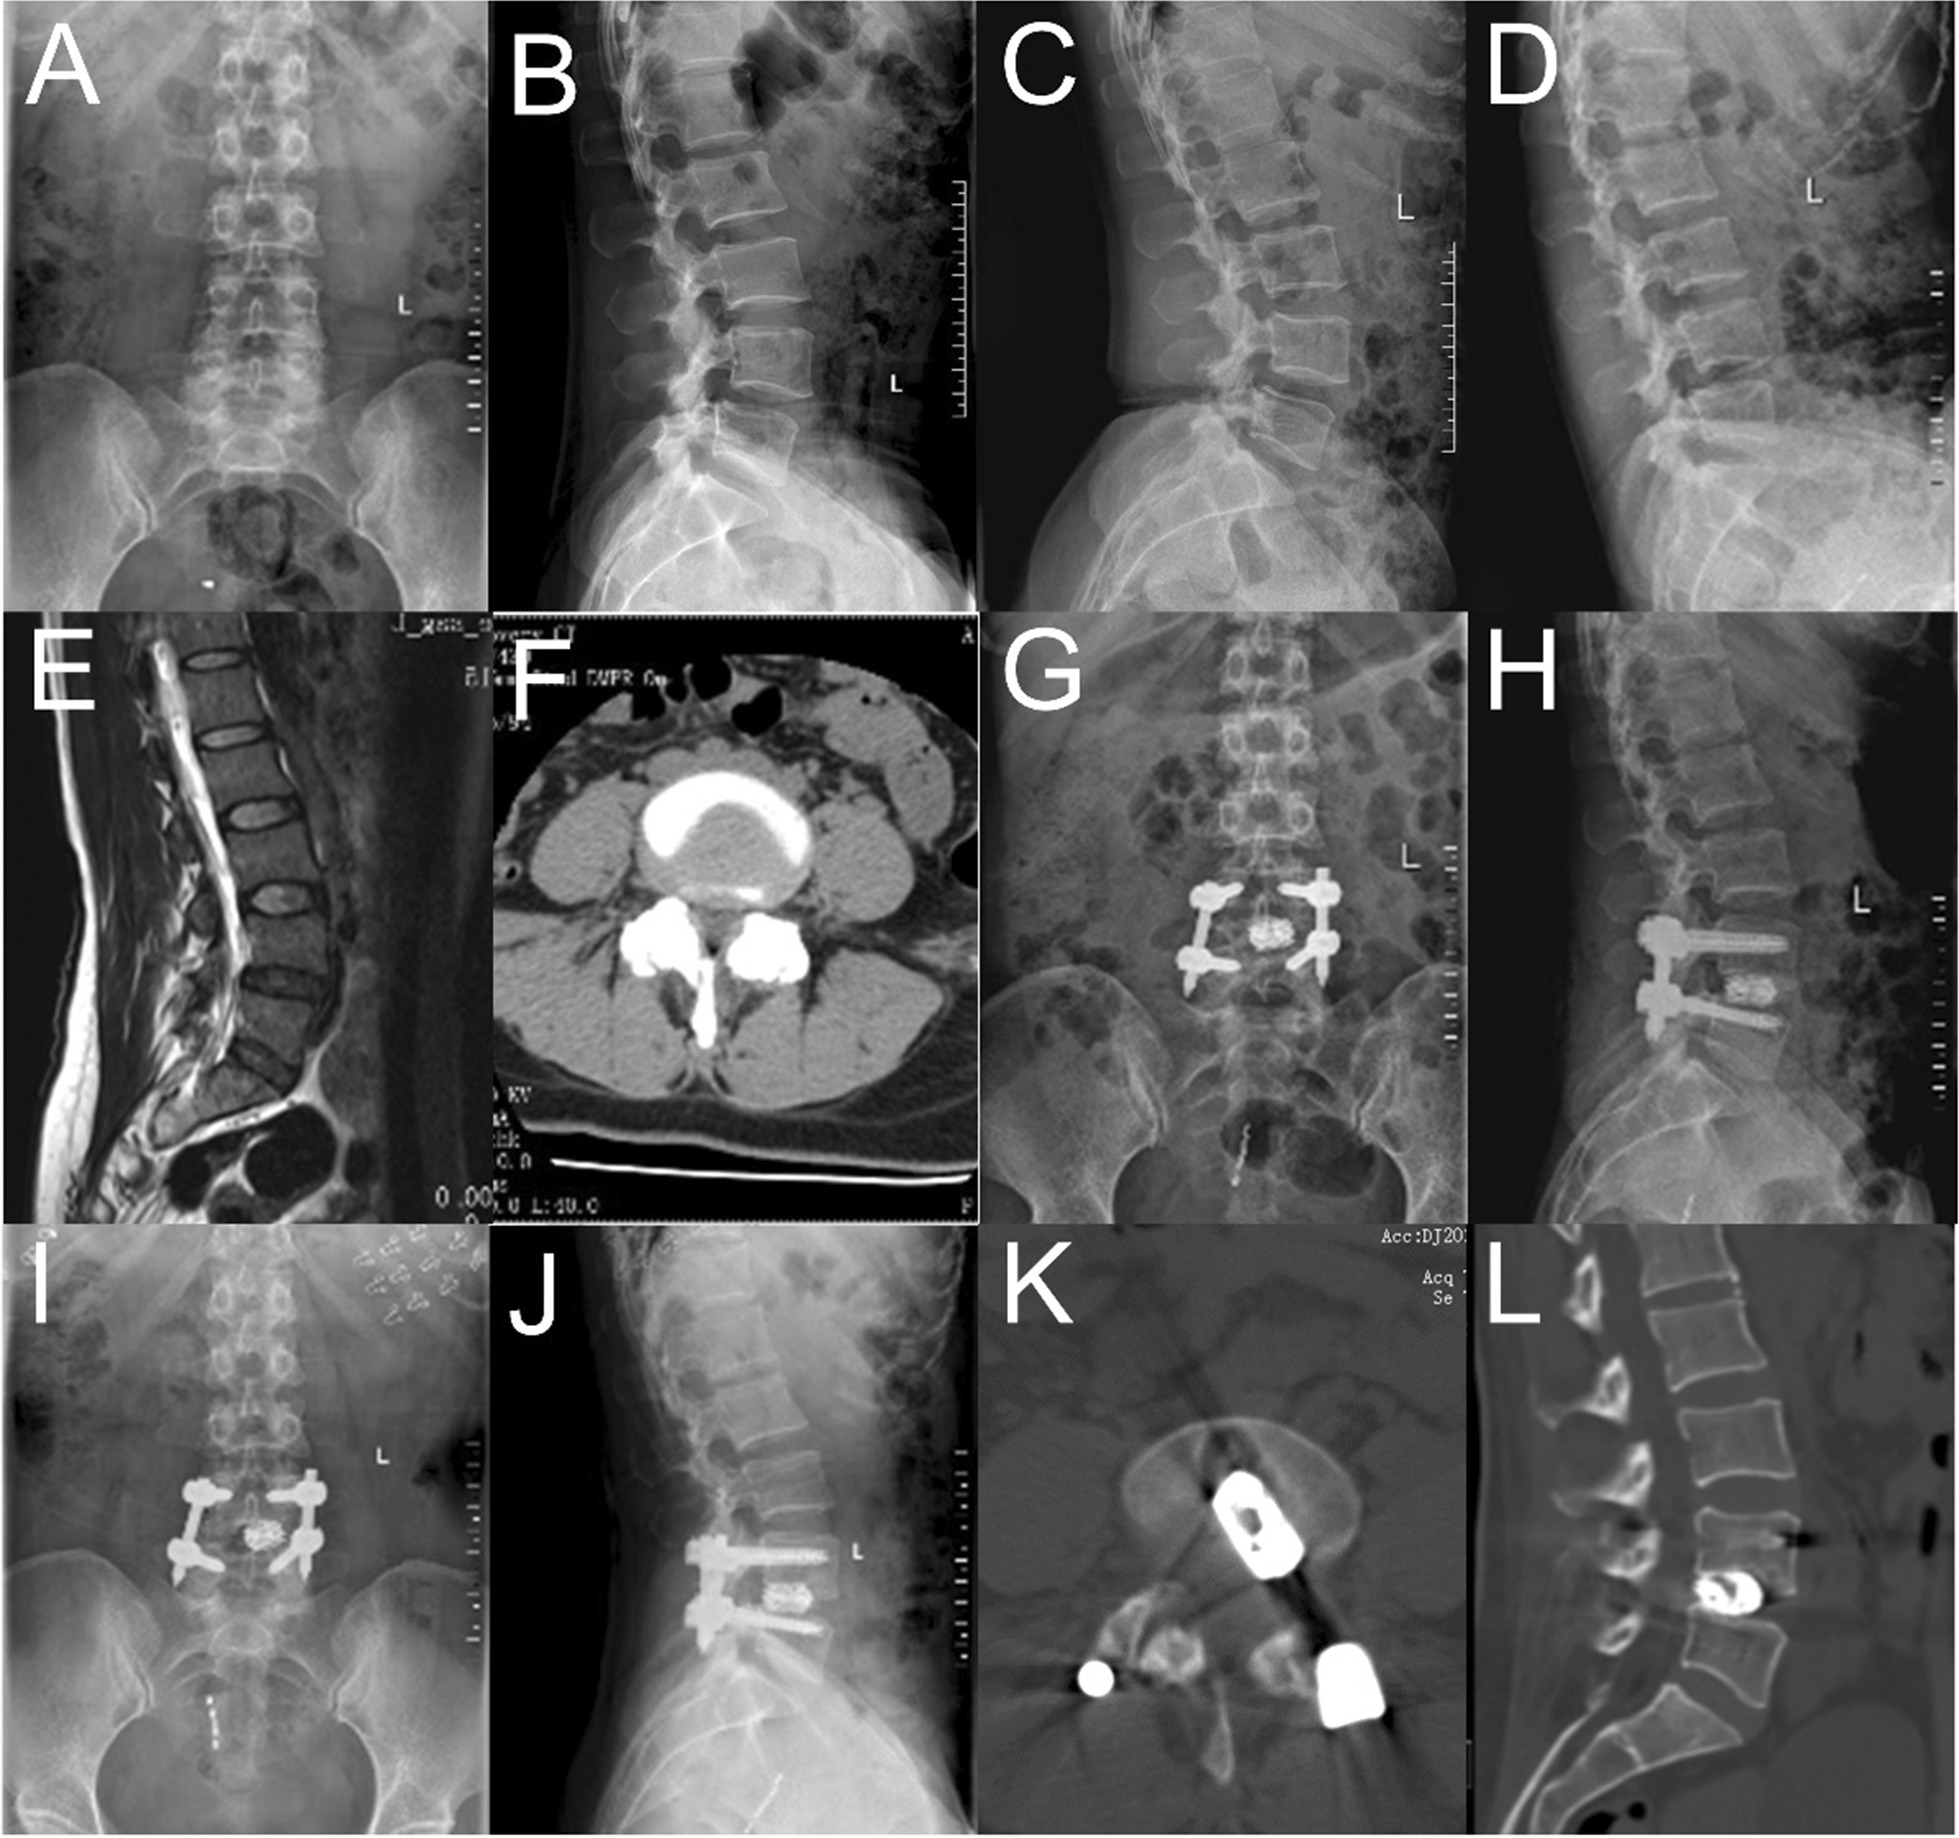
Typical case: A–D are preoperative anteroposterior, lateral, and dynamic X-ray films of the lumbar spine. E is the sagittal plane of preoperative MRI. F is the transverse section of preoperative CT. G and H are anteroposterior and lateral lumbar radiographs at 3 m postop. I and J are anteroposterior and lateral lumbar radiographs at 1 y postop. K and L are CT at the last follow-up
